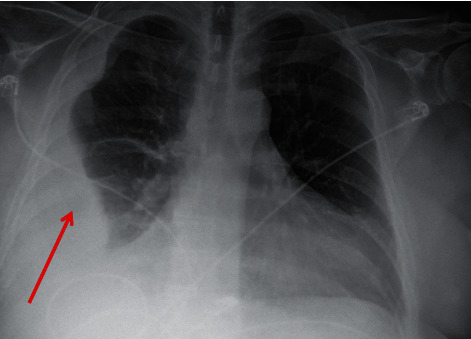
Chest X-ray of the patient upon admission demonstrating right-sided loculated pleural effusion (red arrow).
